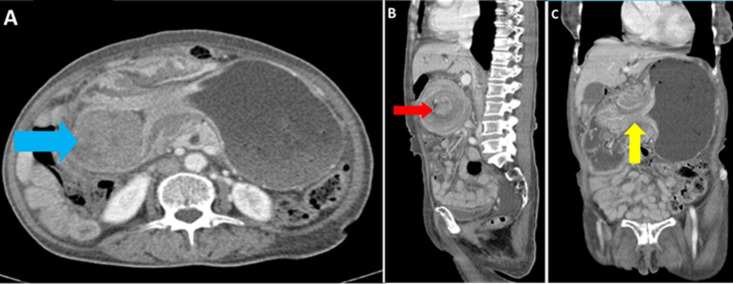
A) CT-scan axial view showing distended stomach with a gastric mass (pathological lead point) (blue arrow); B) saggital view showing ‘target sign’ (red arrow); C) coronal view showing gastroduodenal intussusception (yellow arrow)
